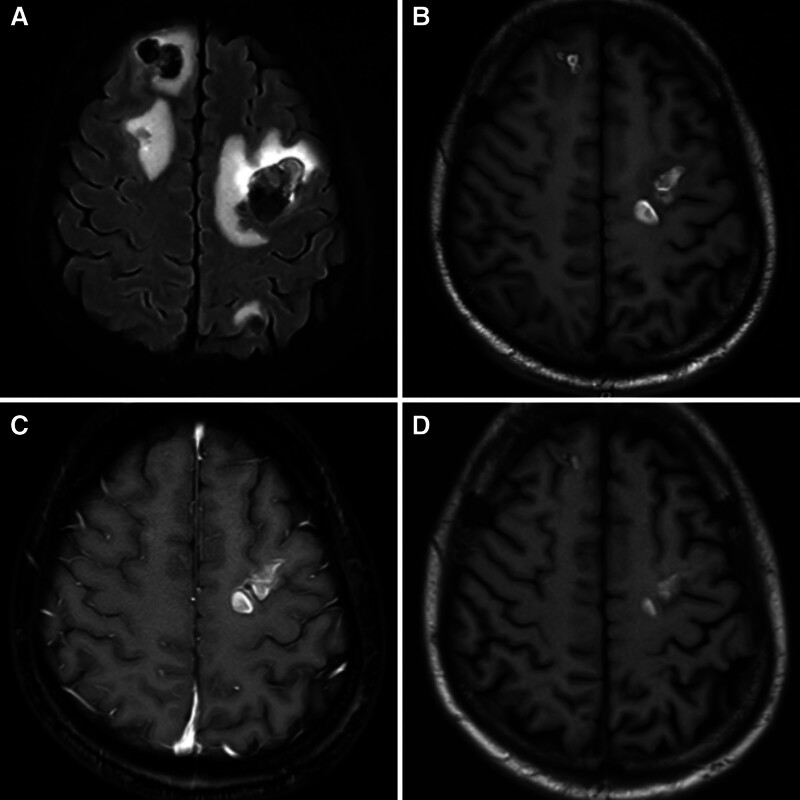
MRI of the brain lesions. (A) Before brain radiotherapy on May 19, 2021. (B) After anlotinib synchronized with brain radiotherapy on July 5, 2021. (C) After 2 cycles of anlotinib synchronized with brain radiotherapy on August 30, 2021. (D) After 4 cycles of anlotinib synchronized with brain radiotherapy on October 24, 2021. MRI = magnetic resonance imaging.
